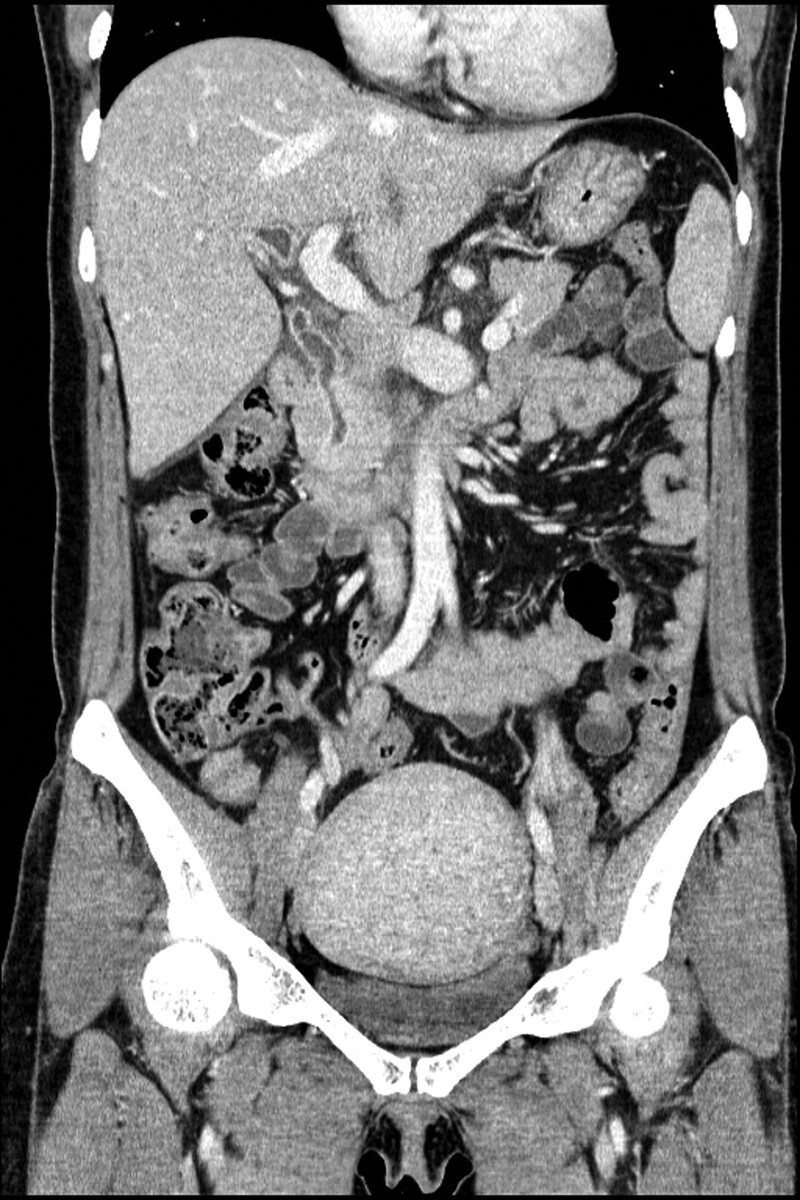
Coronal view of abdominal computed tomography shows mild extrahepatic bile duct dilatation with diffuse wall thickening and enhancement.
